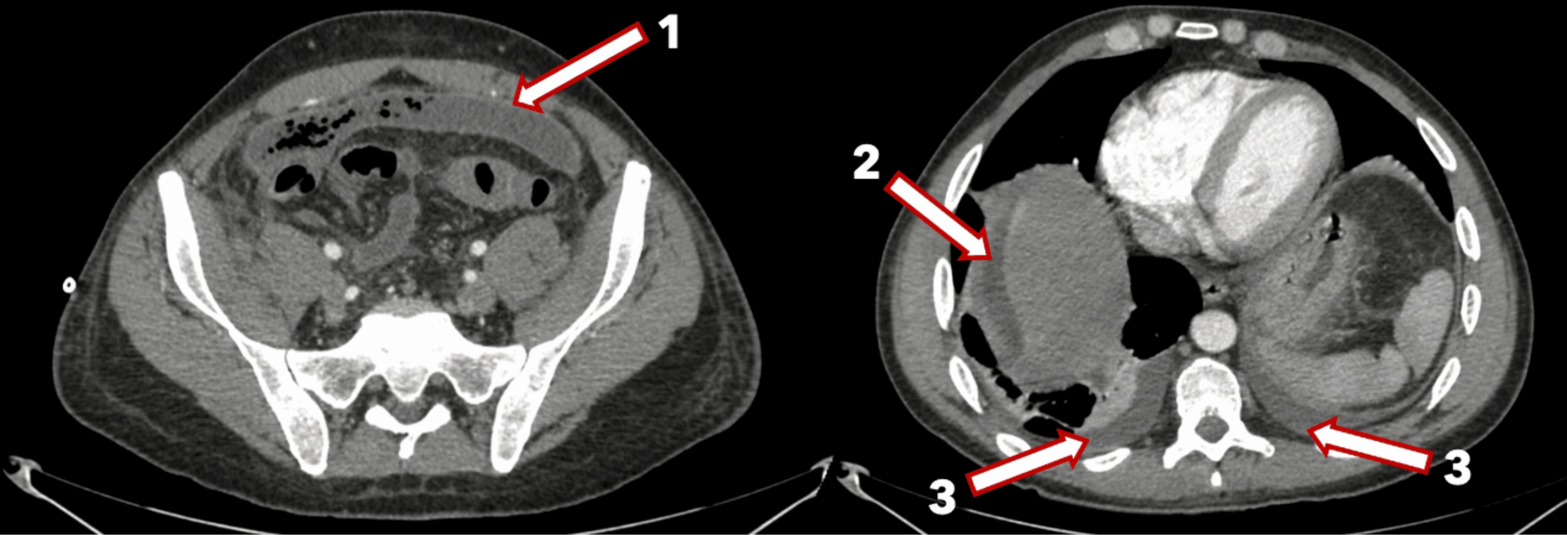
CT abdomen and pelvis demonstrating a reduction in the abscess volume, measuring 5 cm (AP) × 15 cm (TS) (1), with stable right subdiaphragmatic and subhepatic fluid (2) and minimal bilateral pleural effusions (3). White arrows (outlined in red) indicate the described findings. TS: transverse; AP: anteroposterior
